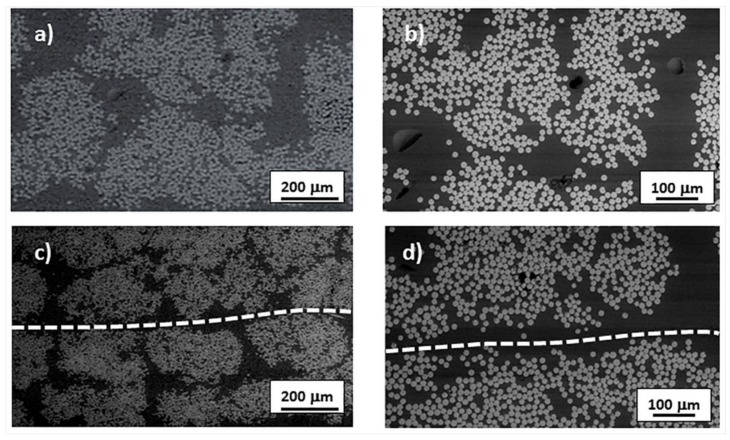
(a,b) Cross section of unconsolidated E-glass/LPET tape as received; (c,d) cross section of two E-glass/LPET tapes after ultrasonic assisted filament winding at 0.18 rad/s.
